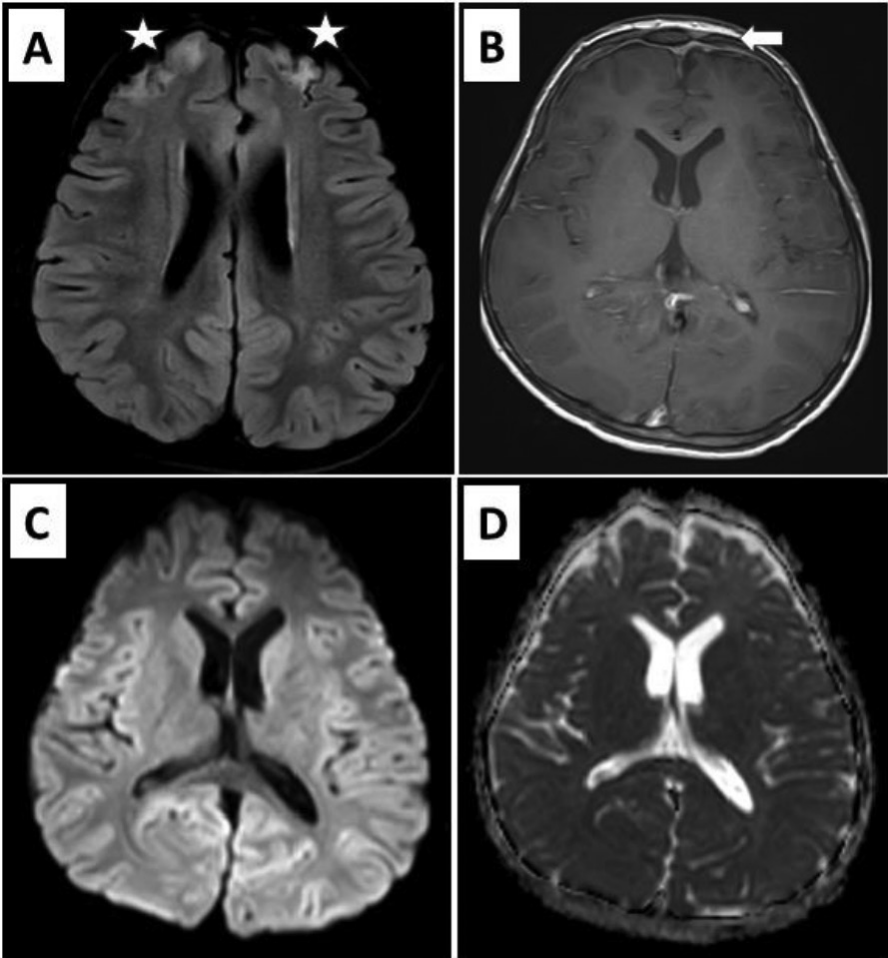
Follow-up MRI images taken after 8 months of an 8-year-old boy with Pott’s puffy tumour showing: A – Axial T2 FLAIR image showing bifrontal cortical foci of increased signal intensities (white stars) suggestive of gliotic changes; B – Axial contrast-enhanced images showing associated smooth pachymeningeal thickening (white arrow); C and D – DWI and ADC images, respectively, showing no features of residual collections
